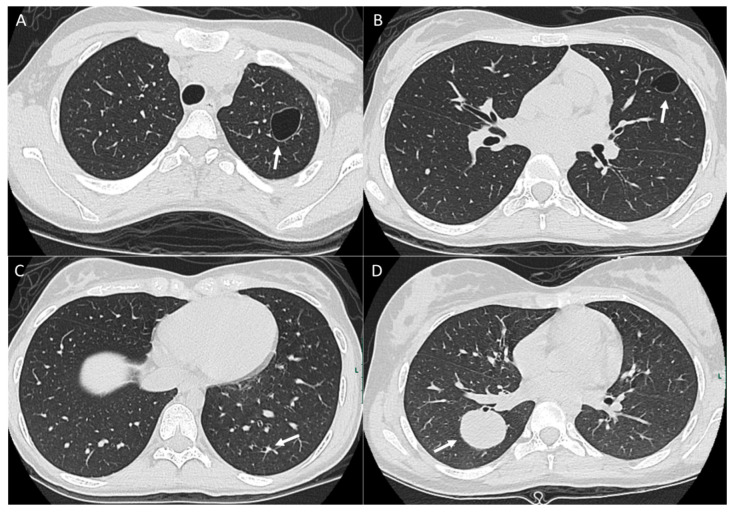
Patient with osteosarcoma and lung metastasis. Axial non-enhanced images (A,B) show cystic lesions in the left upper lobe (arrows). Axial non-enhanced image (C) shows a left lower lobe small cystic lesion with a nodular component (arrow). These cystic lesions were new compared to baseline CT and compatible with cystic metastasis. Axial non-enhanced image (D) from follow-up CT shows interval development of a large right lower lobe metastatic lung mass (arrow).
